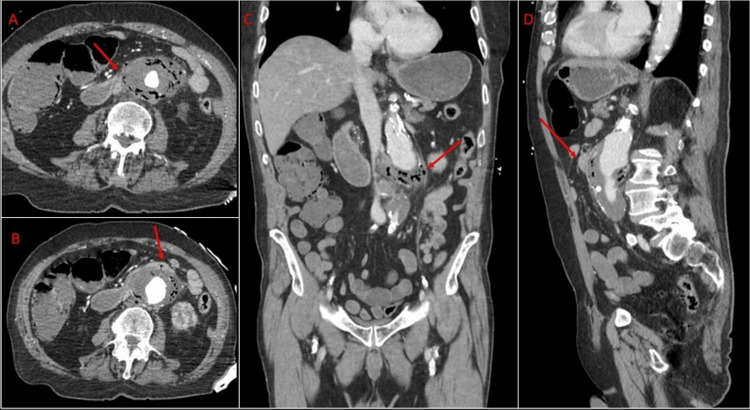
Computed tomography images of Case 1. A: Axial view demonstrating air within the aneurysm sac abutting the third and fourth portions of the duodenum (crossing the aneurysm anteriorly – arrow). B: Axial view shows the fistula tract evidenced by a focus of air extending through the duodenal wall and into the aneurysm sac (arrow). C: Coronal view showing the 6.5-cm infrarenal abdominal aortic aneurysm with air in the aneurysm sac (arrow). D: Sagittal view showing the aneurysm sac abutting the third portion of the duodenum anteriorly (arrow).
